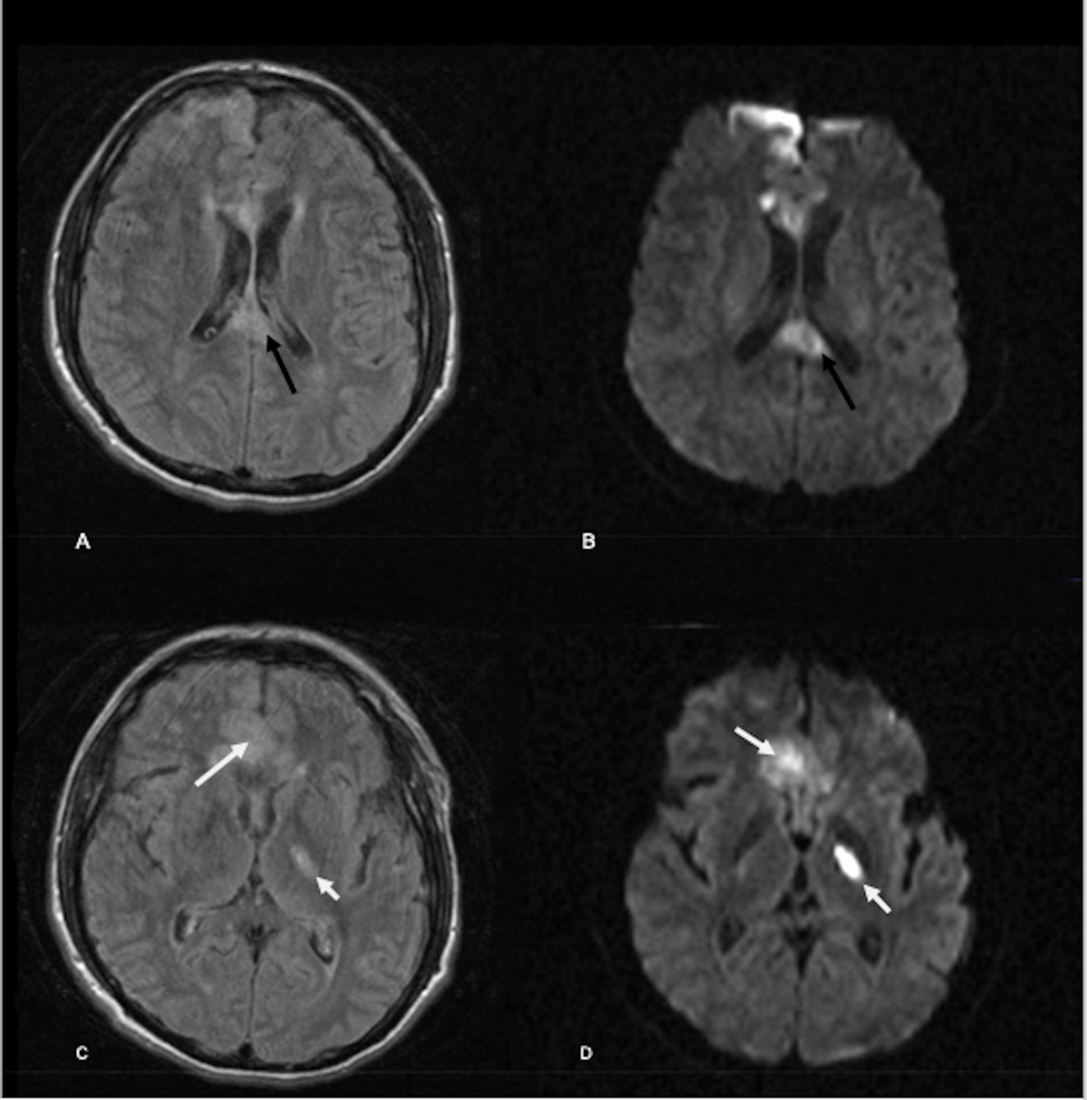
Brain MRI on second admission Axial MRI brain FLAIR and diffusion images on readmission. (A-D) Axial FLAIR and diffusion images demonstrate a slight decrease in the previously visualized restricting corpus callosum lesion (black arrows). However, new FLAIR hyperintense and diffusion restricting lesions are seen in the right para sagittal frontal cortex and left thalamus (white arrows). FLAIR, fluid-attenuated inversion recovery
